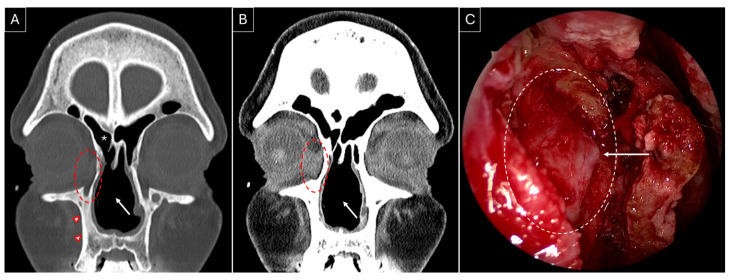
Non-contrasted sinus CT, coronal section, bone (A) and soft tissue (B) windows, showing right-sided dacryocystitis (red dotted circle). The presence of a nasal septal perforation (white arrow), osteitic bone remodelling (red arrowheads), and a previously performed right-sided hemi-modified Lothrop frontal sinusotomy can be noted (white asterisk). (C) Intraoperative endoscopic image of the right lacrimal sac medial wall (white dotted circle) after removal of the overlying lacrimal bone. The white arrow indicates tenting of the medial wall of the right lacrimal sac by the Bowman lacrimal probe.
